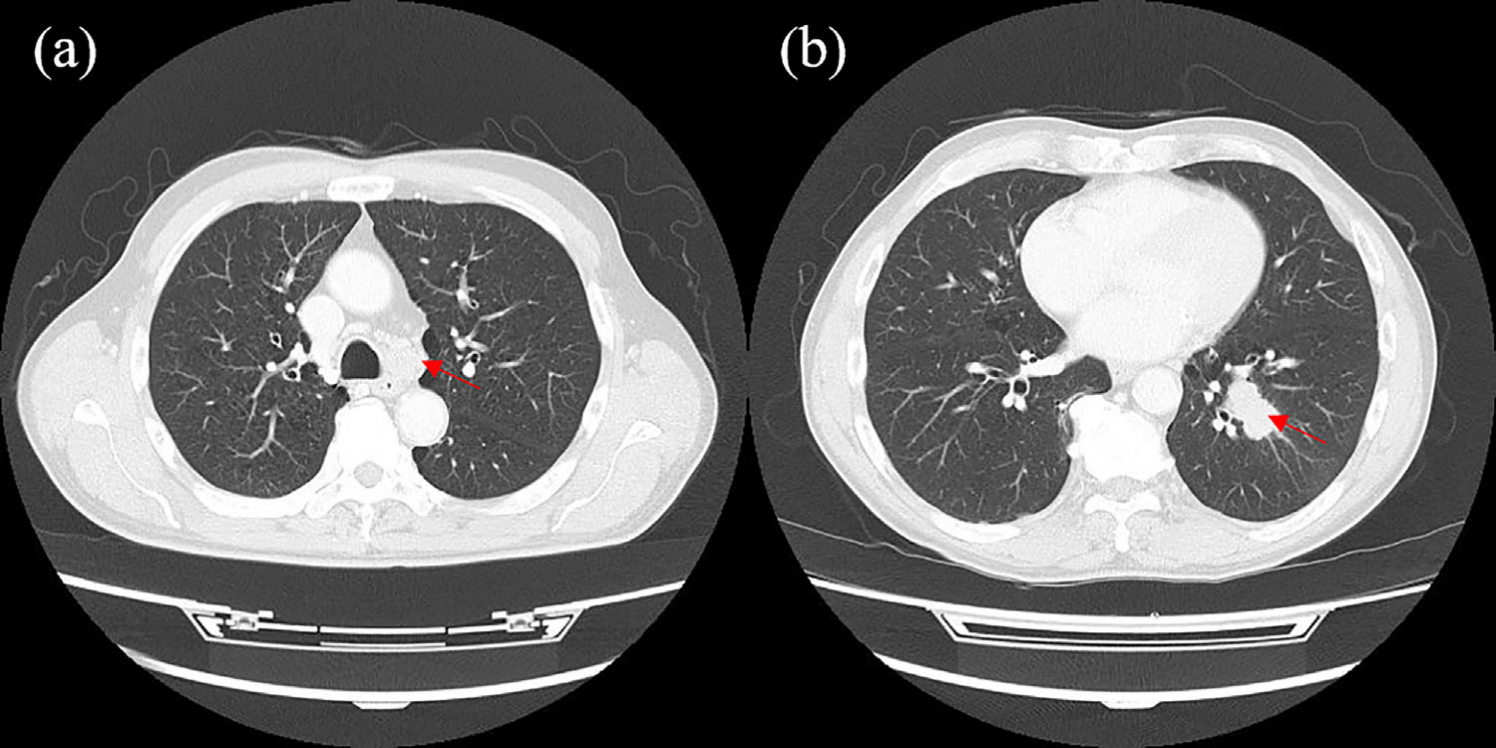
Whole body computed tomography scan. (a) A computed tomography scan shows an ill‐defined mass in the left lower lobe (red arrow). (b) Enlargement of the left hilar lymph nodes (red arrow).
